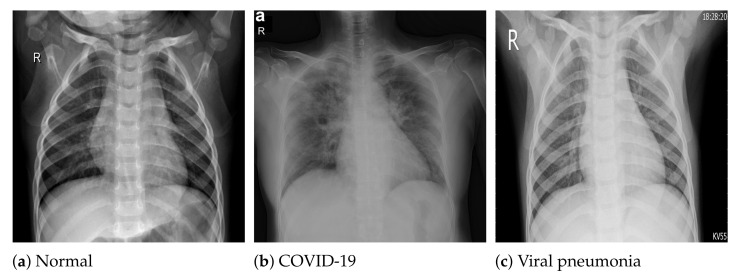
Sample X-ray images from the used dataset.
